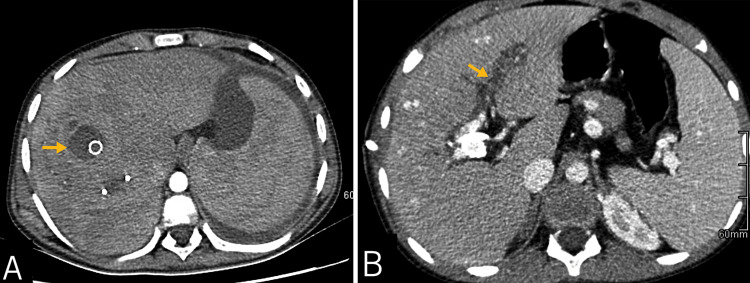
Abdominal contrast-enhanced computed tomography (A) It reveals a low-density collection around the transjugular intrahepatic portosystemic shunt (TIPS) stent (yellow arrow) (B) and dilation of the intrahepatic bile duct on the left side (yellow arrow).
